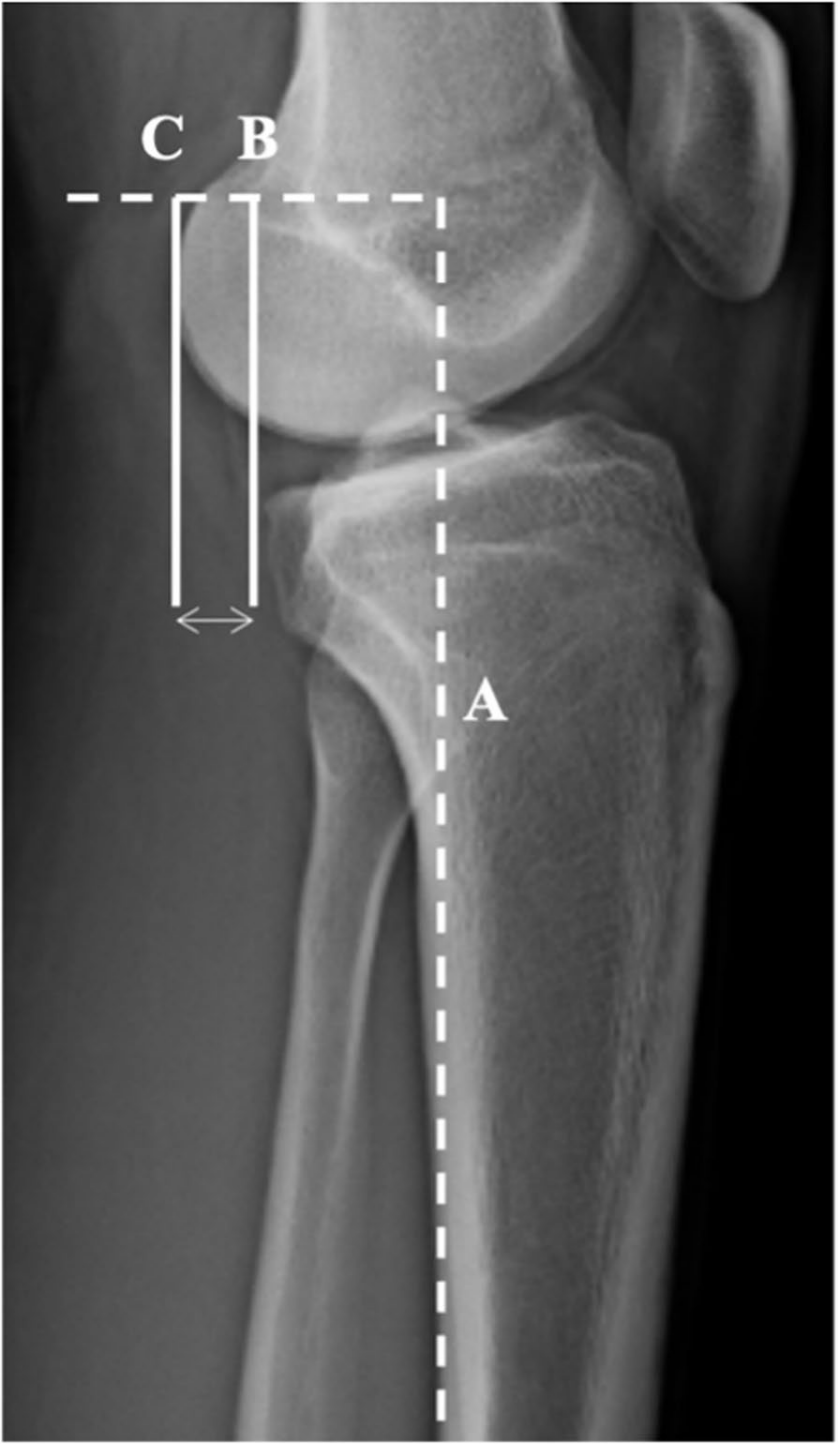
Lateral right knee radiograph demonstrating Static Anterior Tibial Translation (SATT). Measurement of SATT in monopodal WB x-rays. The posterior tibial cortex is the reference (line A). Two lines are traced parallel to line A and. tangent to posterior part of the medial plateau (line B) and medial femoral condyle (line C). SATT is the distance between line B and C
